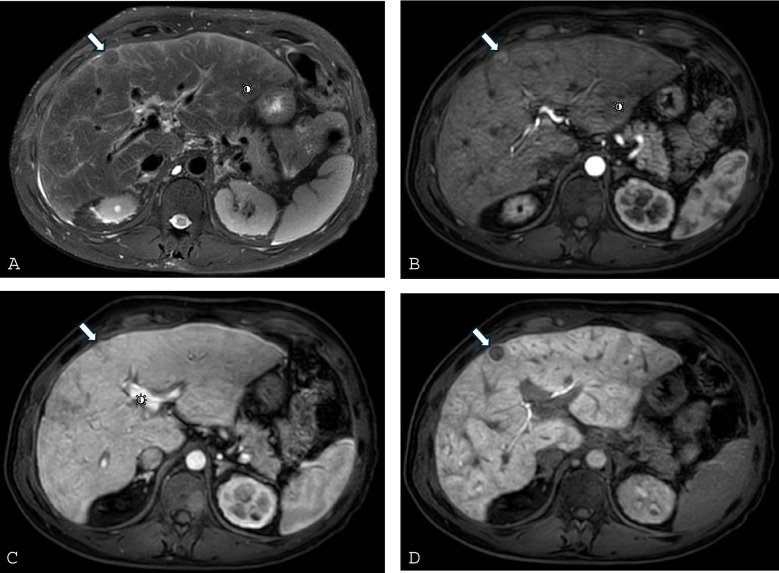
42-year-old male status post Fontan palliation with Fontan associated liver disease. Axial fat-suppressed T2-weighted (A) MR image shows peripheral T2-hyperintense reticulation typical of FALD representing sequalae of hepatic congestion and fibrosis. A lesion is present in the anterior right hepatic lobe (*arrow*) that is T2-hypointense. Dynamic post-contrast fat-suppressed T1-weighted MR imaging during the arterial phase (B) shows arterial phase hyperenhancement with subsequent washout on the portal venous phase (C). The lesion does not retain the hepatobiliary contrast agent on the hepatobiliary phase (D) but does have a thin rim of peripheral retention. The lesion was biopsied and proven to be HCC
